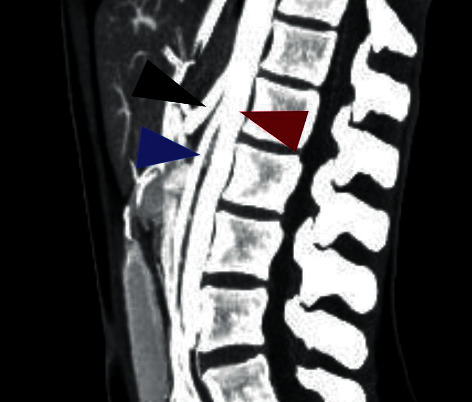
CT angiography revealing severe acute angularity of the SMA-aortic take off. Black arrow: celiac artery; maroon arrow: aorta; blue arrow: superior mesenteric artery.
